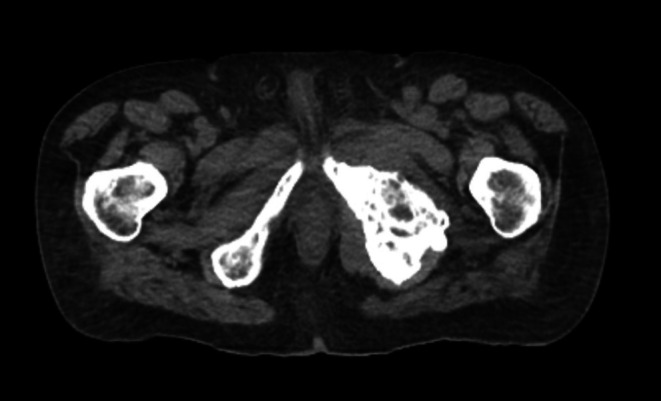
Pre admission CT scan demonstrating pelvic structural abnormality.
